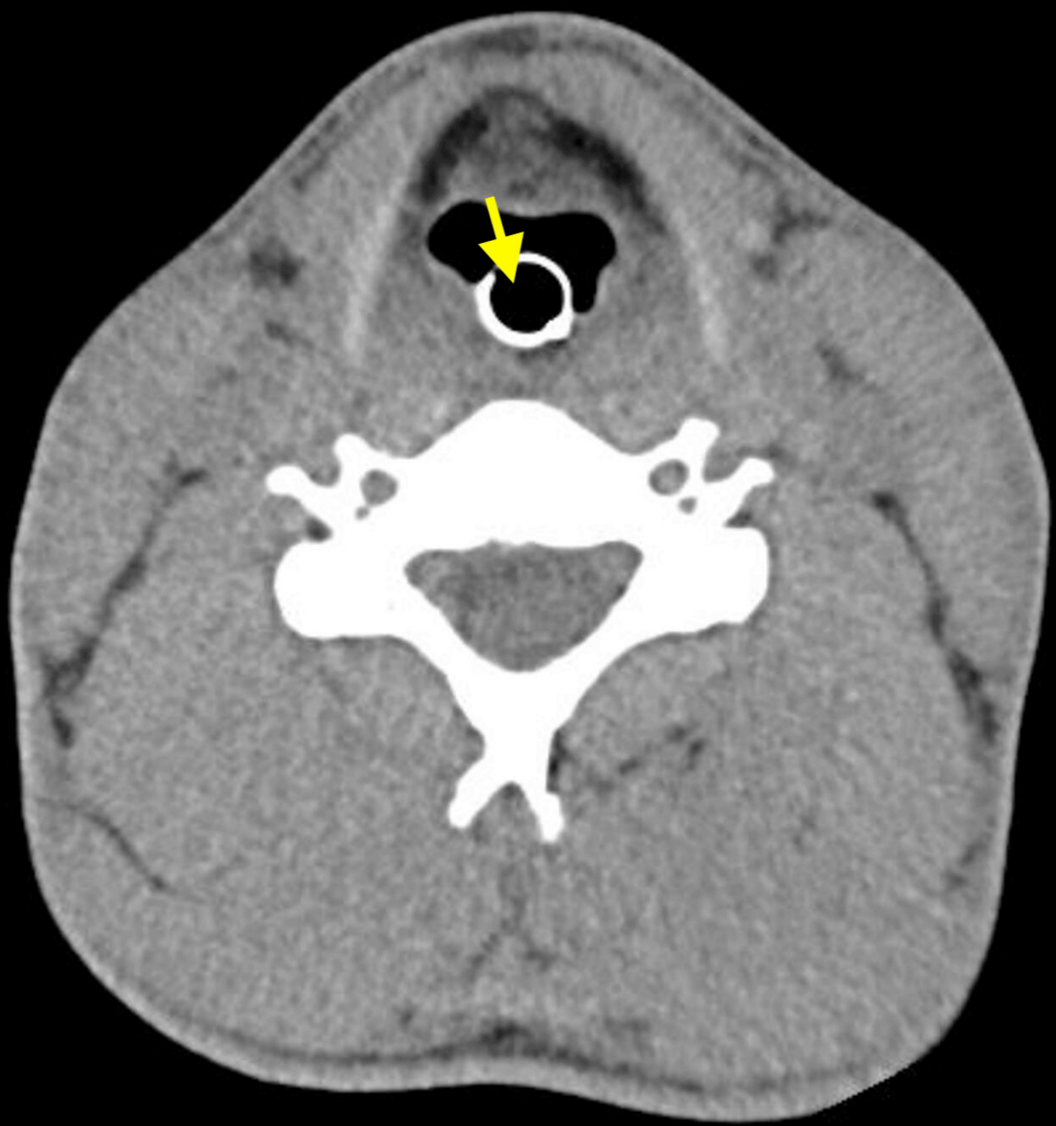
Neck CT scan of the second patient (LB) Axial image of a neck CT scan without contrast, localized at the C5 level. No abnormalities are found, rather for the presence of an orotracheal tube inside the trachea (yellow arrow). The cervical vertebra is preserved, without signs of fracture or trauma. There are no signs of neck hematoma. CT: computed tomography
